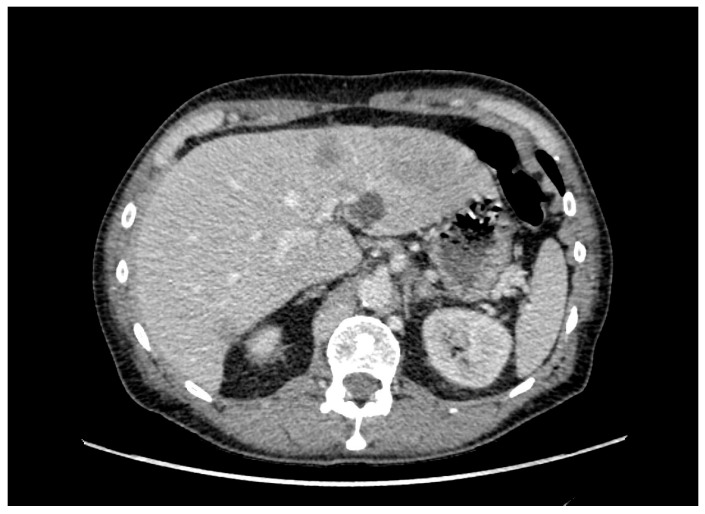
Imaging characteristics of metastatic progression of urothelial carcinoma on computed tomography (CT) of the abdomen.
